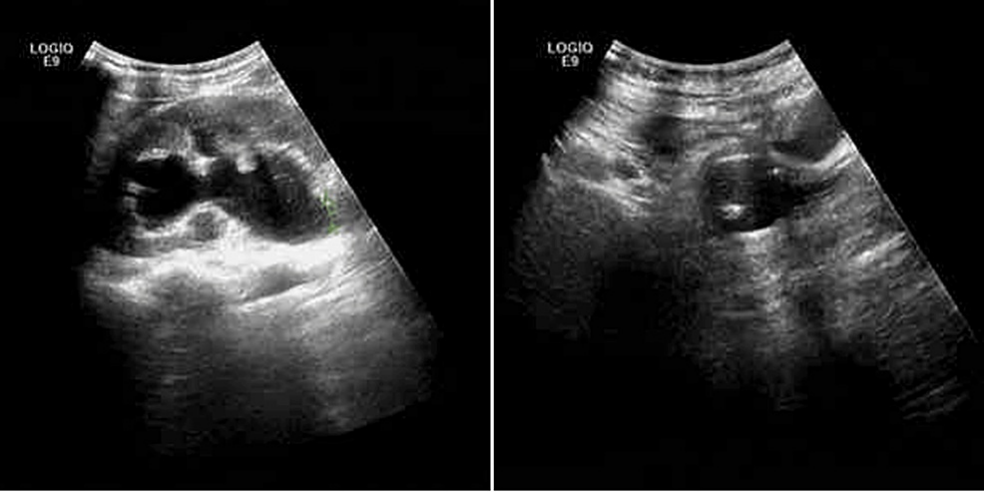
Ultrasound findings reveal a separation in the renal pelvis of the left kidney and dilation of the left ureter's upper segment.
